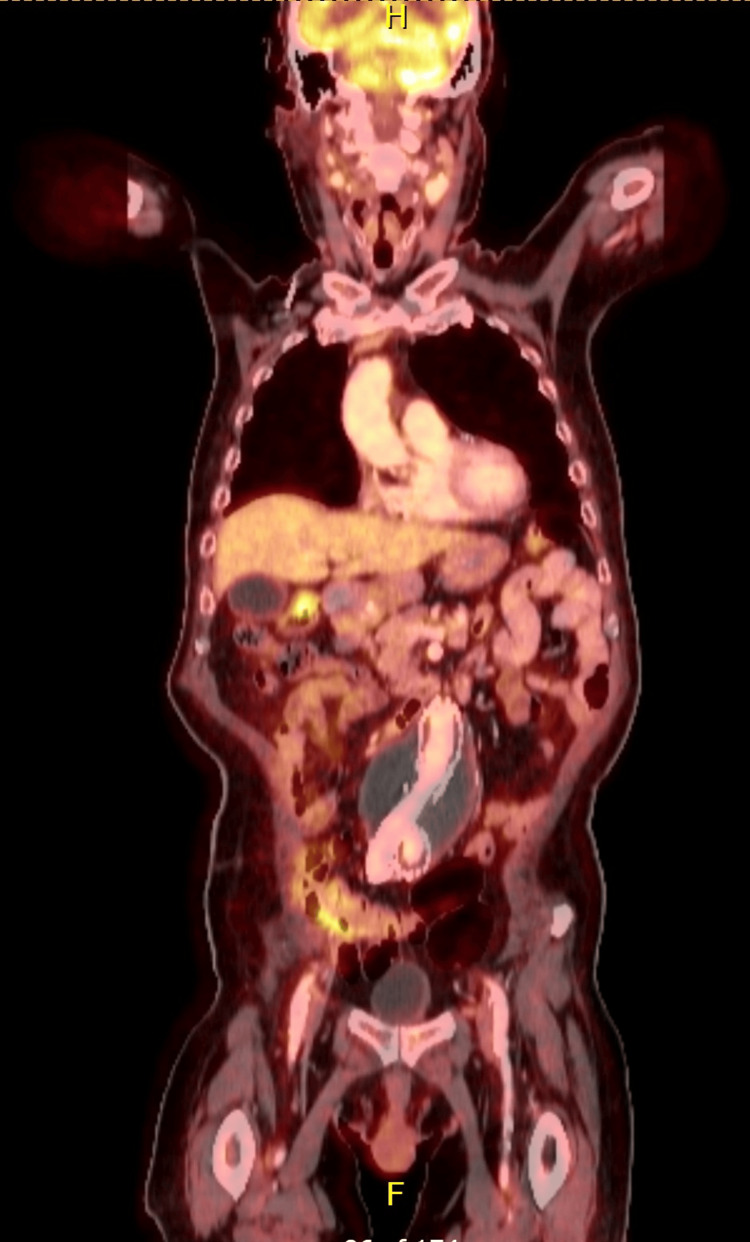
CT/PET with complete remission after treatment
